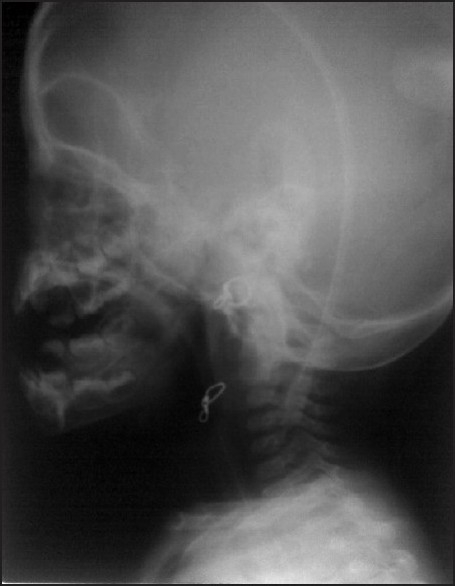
Postoperative neck X-ray lateral view
